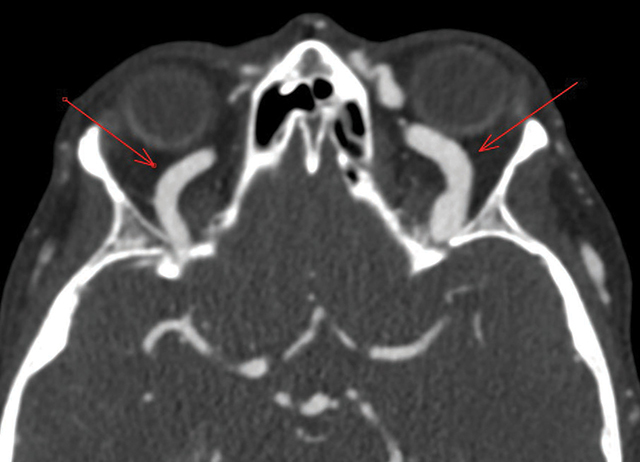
CT angiography showing dilatation of the superior ophthalmic veins.
